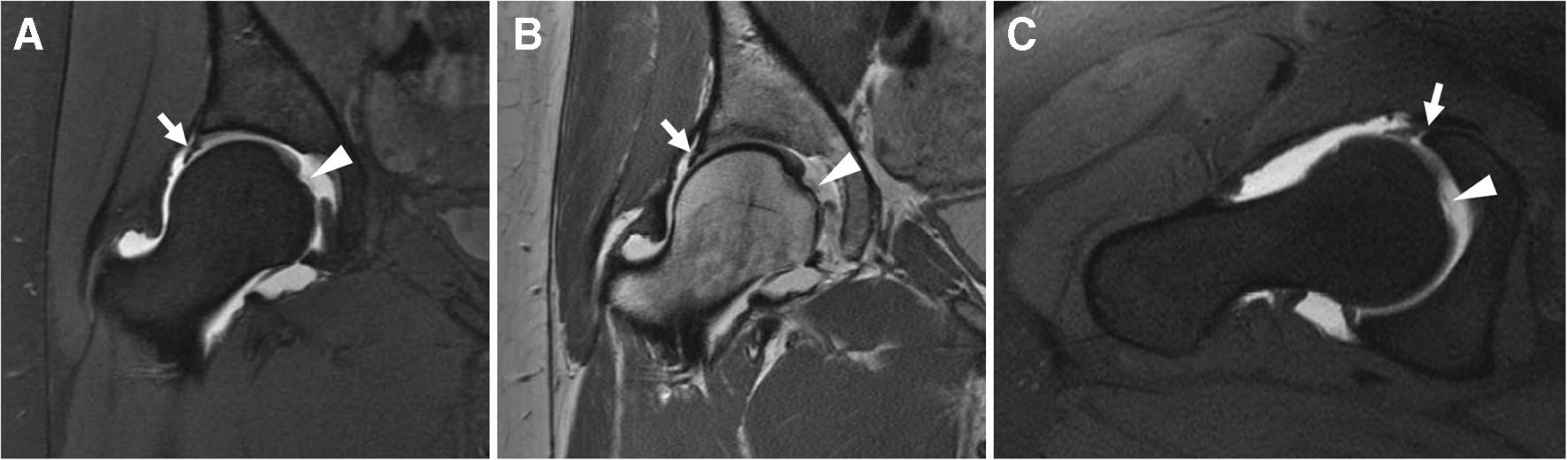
Ligamentum teres tear. Twenty-eight-year-old ex-ballerina with chronic hip pain. Coronal T1-weighted fat-suppressed (A), coronal intermediate-weighted (B), and axial oblique T1-weighted fat-suppressed MR arthrogram images show chronic tearing and resorption of the ligamentum teres (arrowheads) as well as a torn labrum (arrows)
